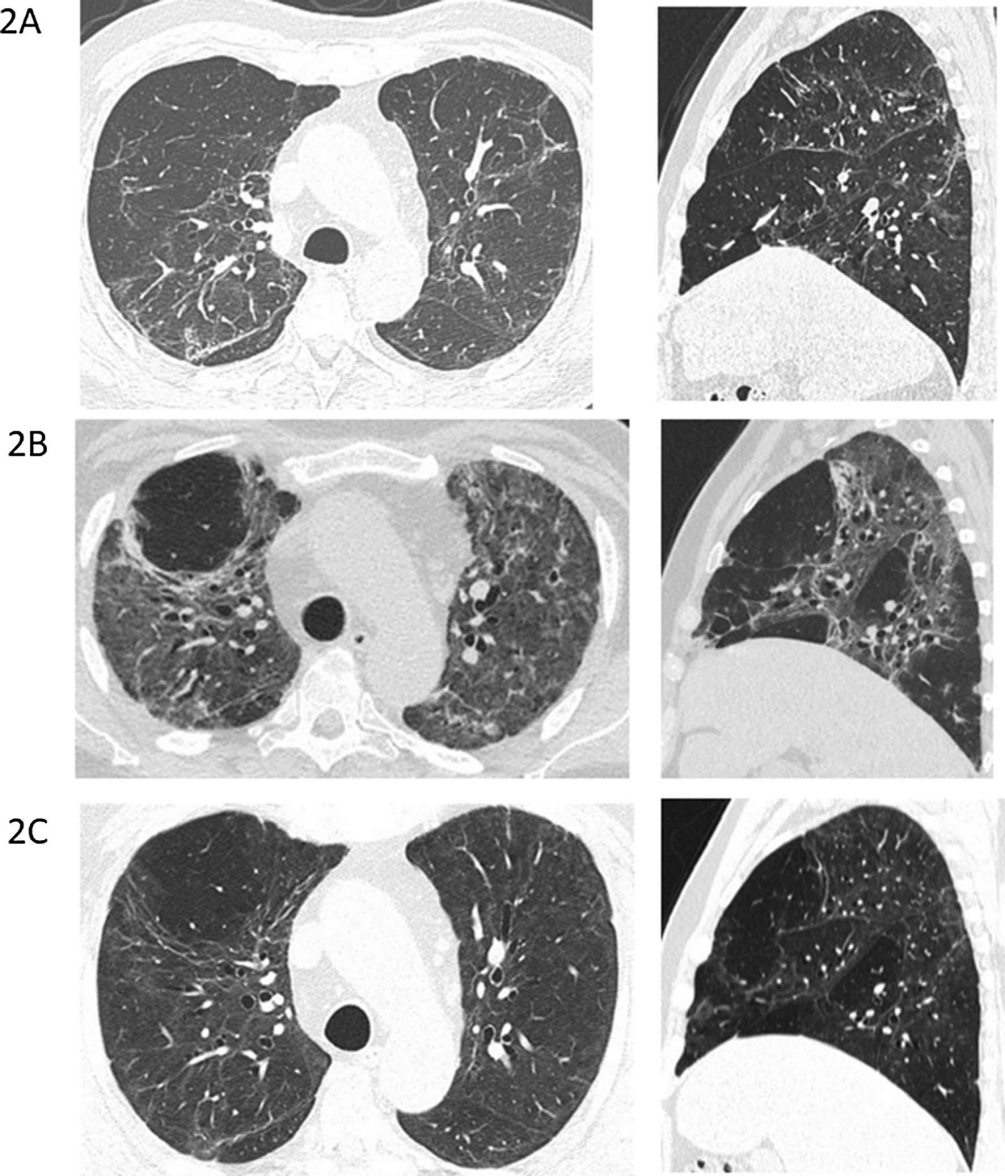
A CT scan at the end of the H1N1 infection with ground-glass opacities, reticulations, and traction bronchiectasis with upper region dominance. B CT scan 1 month after onset of COVID-19 infection showing a large extent of ground-glass opacities in both upper and lower regions, with a sparring of the subpleural regions. There is volume loss and bronchiectasis, all suggesting ongoing infection and inflammation with a component of organizing pneumonia. C CT scan 5 months after COVID-19 onset with almost normalization to the level of post-H1N1 infection
